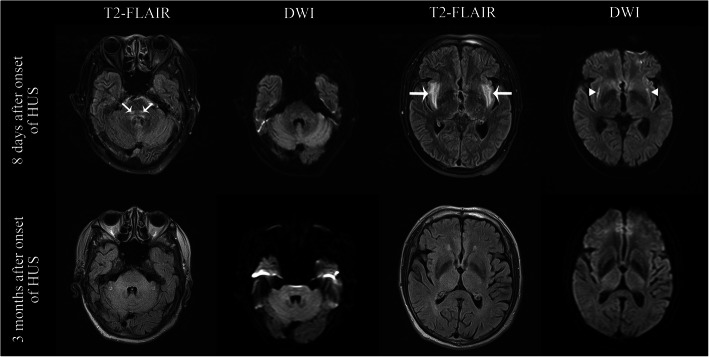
Cranial Magnetic Resonance Imaging (MRI). Bilateral hyperintensities were observed in dorsal brainstem, insula, and external capsule (arrows) on T2 weighted fluid-attenuated inversion recovery (T2-FLAIR) 8 days after onset of confusion (upper panel). The lesions within external capsule also displayed hyperintensities on diffusion weighted imaging (DWI) (triangles). Signal alterations normalized on MRI performed 3 months later (lower panel)
